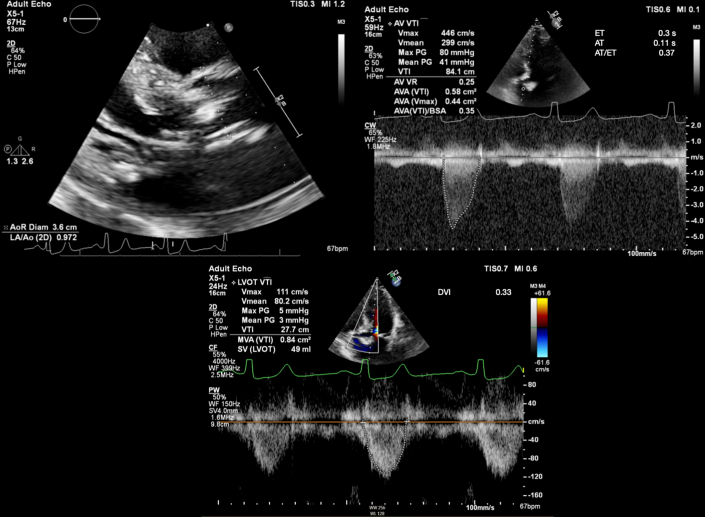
Initial Transthoracic Echocardiography Images Transthoracic echocardiography images displaying severe bioprosthetic aortic stenosis.
